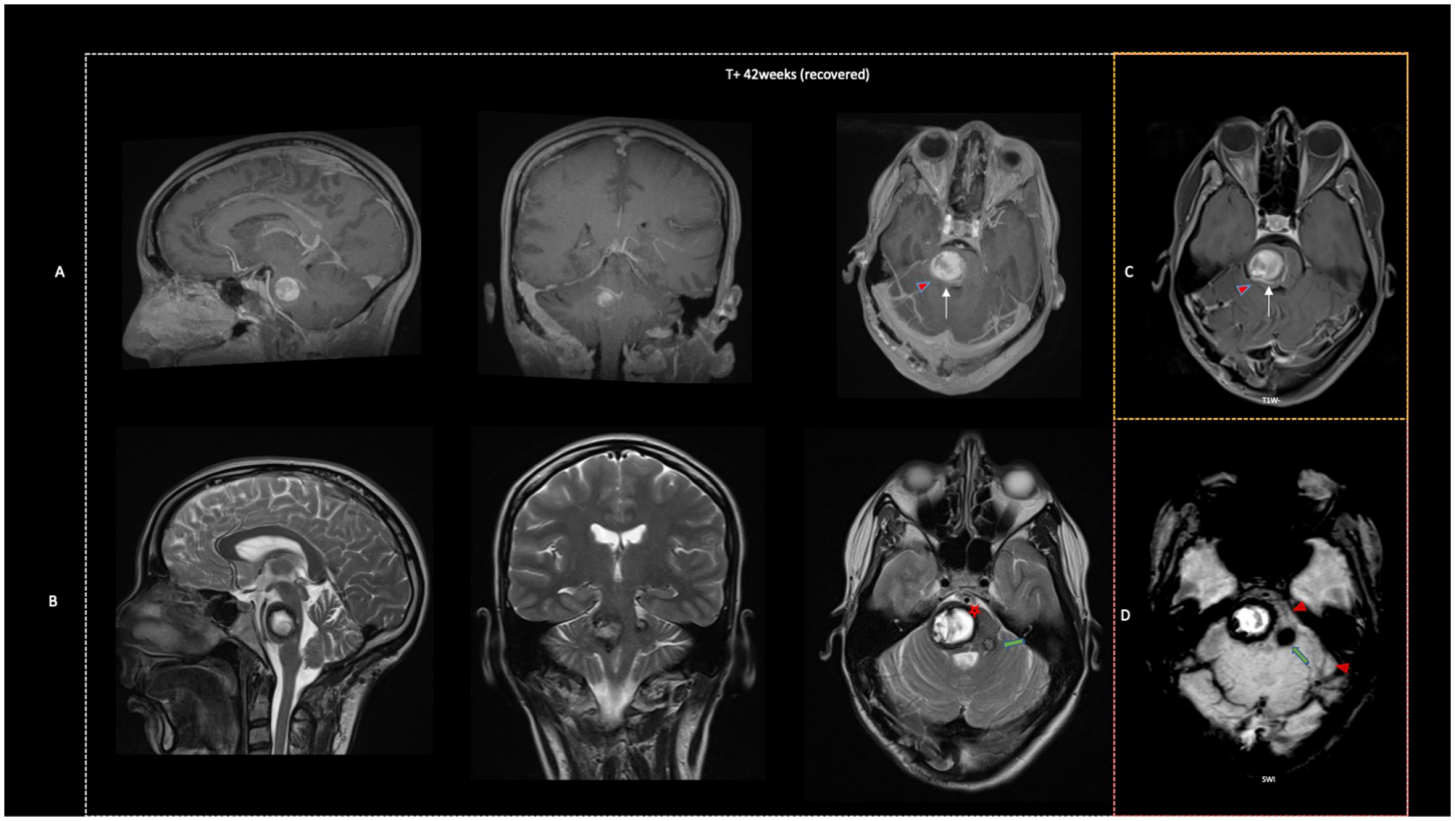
Improvement at 6 months. Sagittal, coronal, and axial MRI: (A) T1W + Gad; (B) T2W axial only: (C) T1W; and (D) SWI. Signal characteristics consistent with late-stage subacute hematoma or extracellular meth-Hb are seen as persisting T1W and T2W hyperintense signals (black star) in the surgical cavity. The additional appearance of a thick hemosiderin ring with hypointense T1, hypointense T2 signal (red star), and clear evidence of thrombus (T1W hyperintensity with reduced filling on T1W + gad) within the DVA system on the right (red arrowhead). The target CCM is confirmed as no longer present on T2* and SWI. The contralateral CCM (green arrow) remains.
